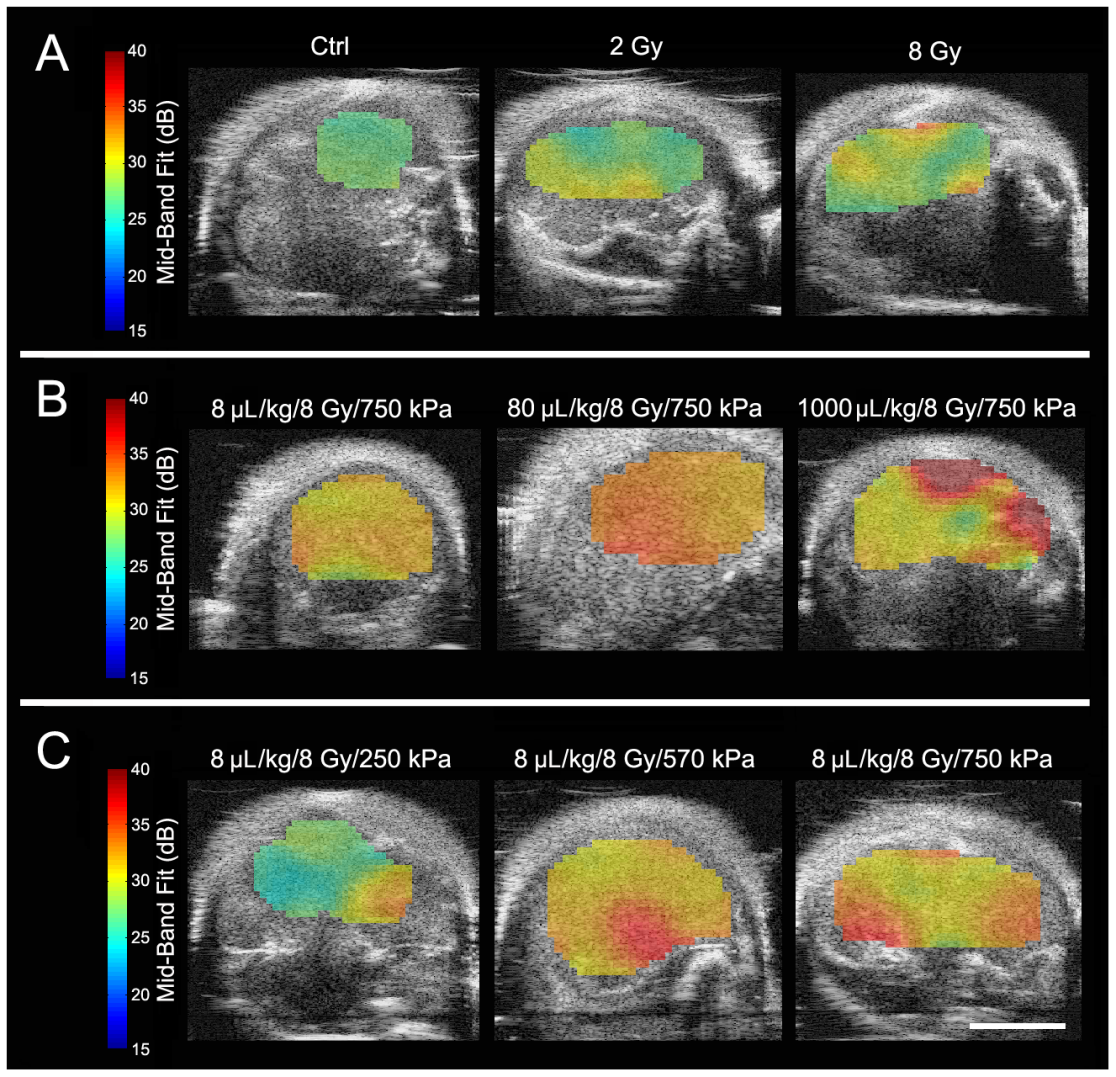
High frequency ultrasound B-mode images with ROI parametric overlays of the mid-band fit biomarker for PC-3 xenografts. (A) Tumors treated with varying radiation doses (0–8 Gy) without ultrasound-microbubble treatment. (B) Tumors treated with varying microbubble concentrations (8–1000 µL/kg) combined with 750 kPa of ultrasound pulse and 8 Gy-radiation. (C) Tumors treated with varying ultrasound pressures (250–750 kPa) combined with 8 µL/kg of microbubbles and 8 Gy-radiation. The scale bar represents 4 mm.
